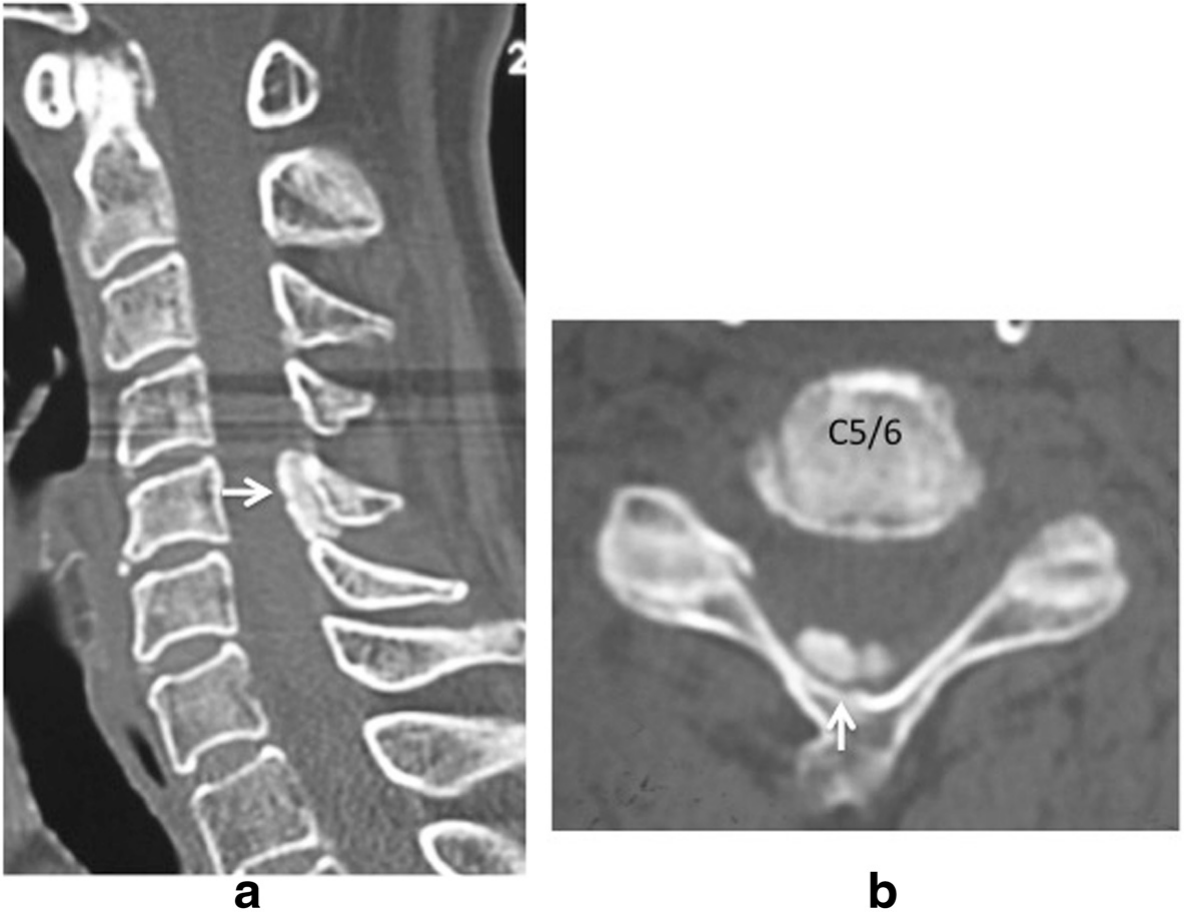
Computed tomography on the second admission. Amorphous high-density area at interlaminar space of C5-C6 (*white arrow*) is seen on sagittal image (a) and axial image (b)
